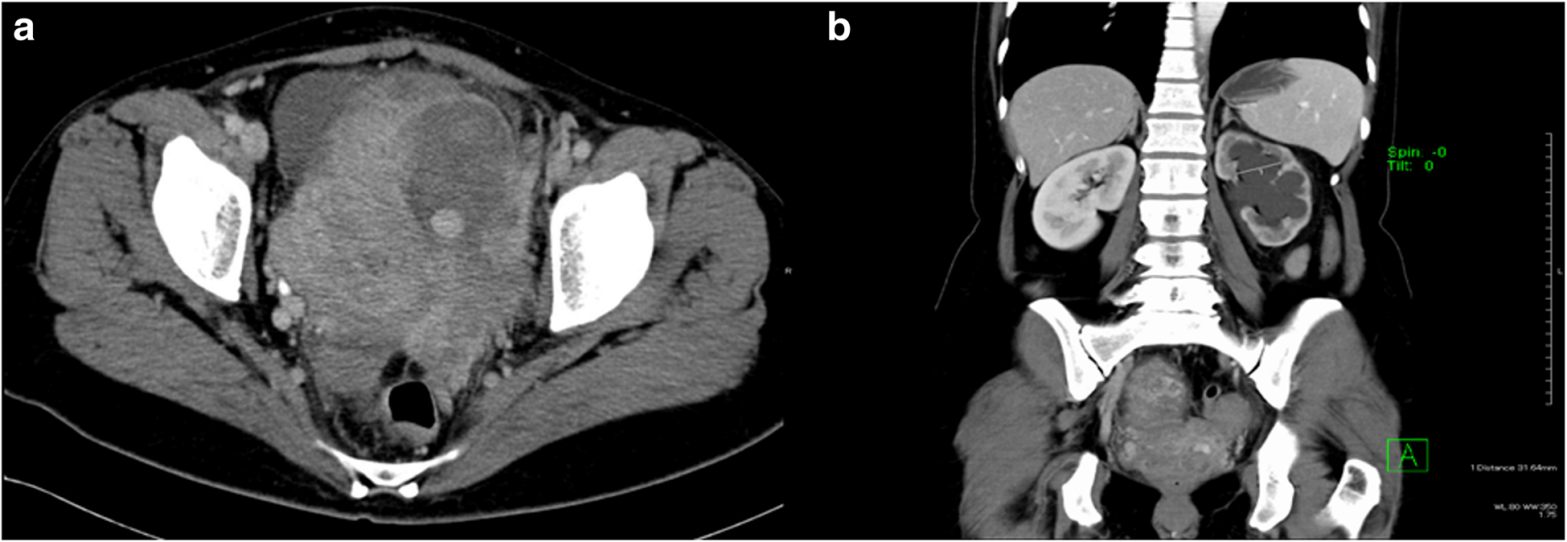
Computed tomography scan demonstrating (a) uterine artery pseudoaneurysm and (b) severe left hydronephrosis (calyceal diameter was over 31 mm)
